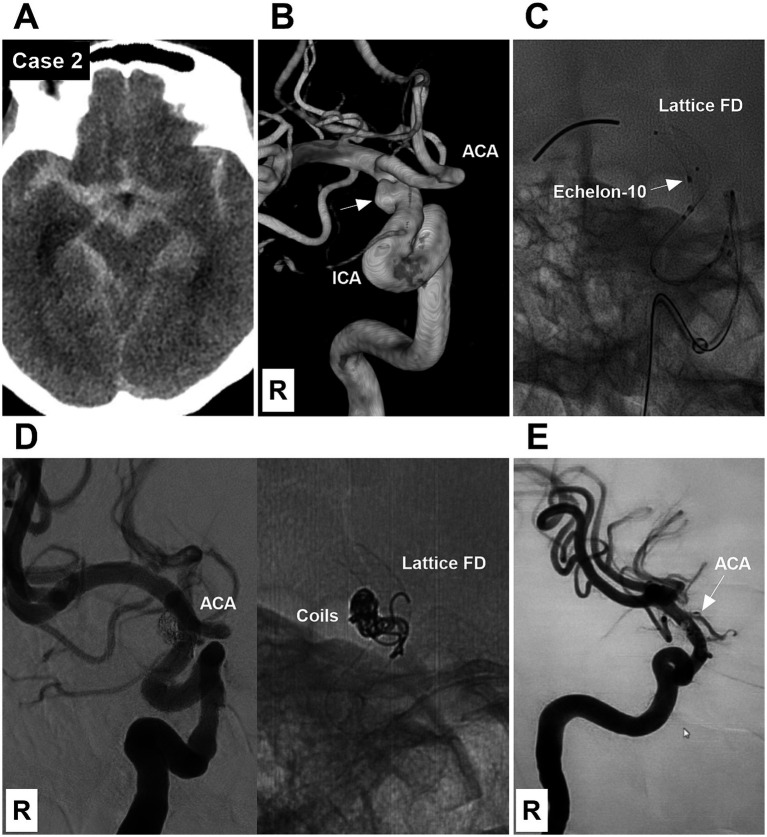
Case 2: The patient was treated by both coiling and deploying a Lattice FD device (Type 2 EVT). (A) Computed tomography showing diffuse subarachnoid hemorrhage of the suprasellar cistern. (B) DSA showing a Type II BBA (arrow) of the right supraclinoid ICA. (C) X-ray image showing an Echelon-10 microcatheter tip (arrow) at the BBA neck and a partially deployed Lattice FD device beyond the BBA neck. (D) Left panel: Postoperative DSA showing the BBA embolized. Right panel: X-ray image showing the coils and Lattice FD device. (E) Six-month follow-up DSA showing that the BBA was cured and the supraclinoid ICA was repaired. However, the right ACA (arrow), covered by the FD, was nearly occluded. ACA, anterior cerebral artery; BBA, blood blister-like aneurysm; DSA, digital subtraction angiography; EVT, endovascular treatment; FD, flow diverter; ICA, internal carotid artery; R, right.
